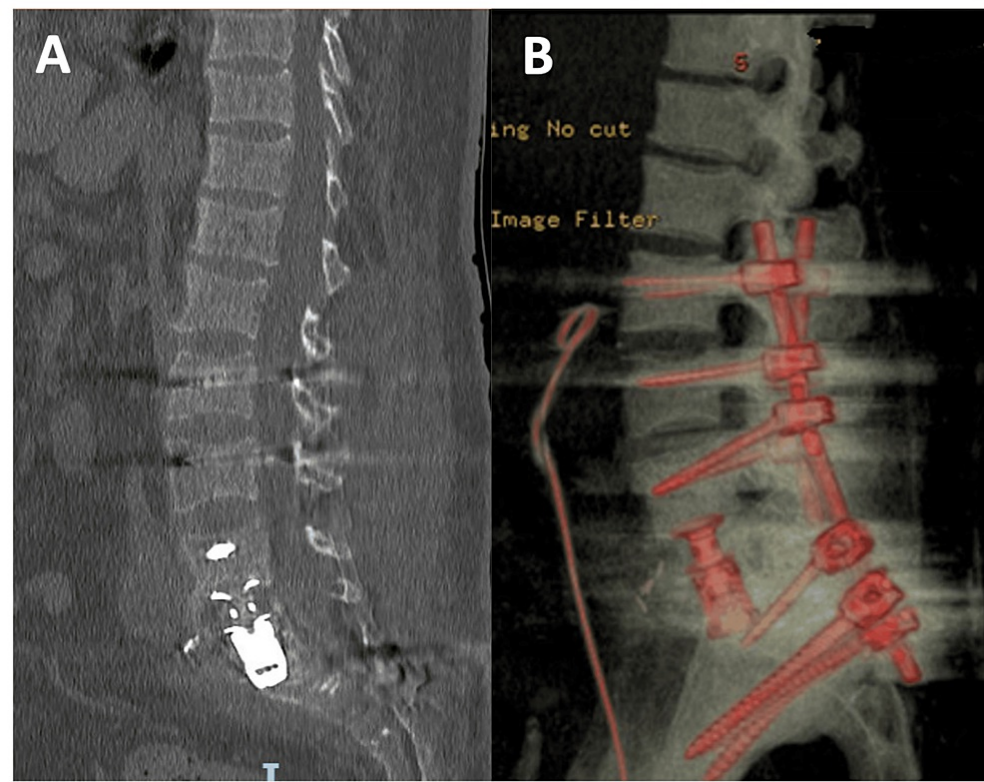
Postoperative imaging First-day postoperative sagittal midline CT scan (A) and 3D reconstruction (B) of the construct. The construct is composed of bilateral pedicular screws L2-L4, with pelvic instrumentation with iliac screws and S2AI screws bilaterally. S2AI, S2 Alar Iliac; CT, computer tomography
